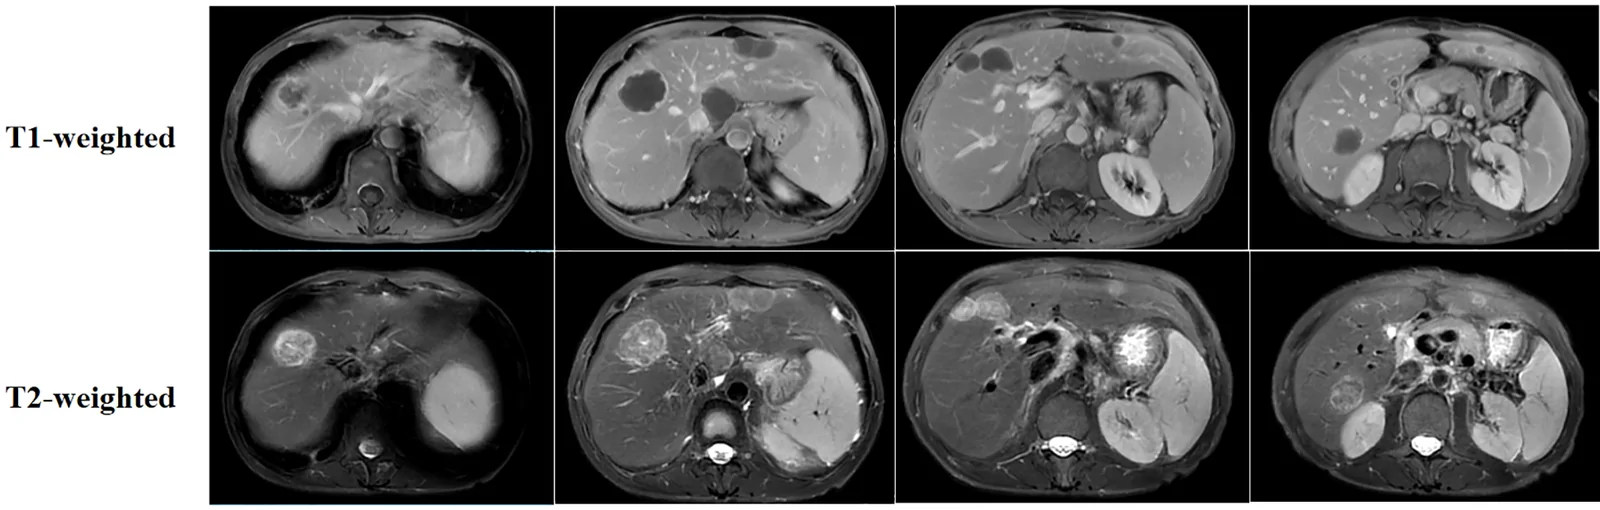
Liver magnetic resonance imaging before surgery.
